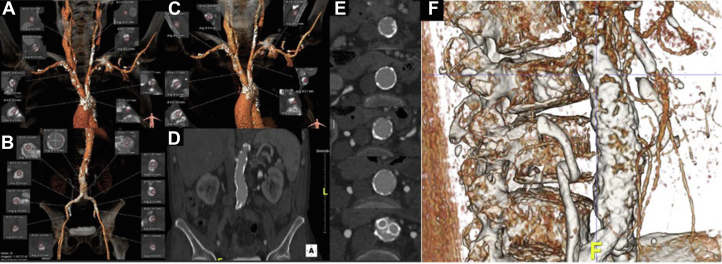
Preoperative Vascular Imaging Preoperative computed tomography angiography with 3-dimensional reconstruction of the (A) common carotid artery, (B) iliofemoral artery, and (C) subclavian artery exhibiting narrow internal diameters. Heavy continuous calcification of the descending aorta is shown on coronal (D) and axial (E) preoperative computed tomography angiography imaging. The lateral view of the descending aorta on 3-dimensional reconstruction shows lack of an adequate calcium-free window for transcaval access (F).
